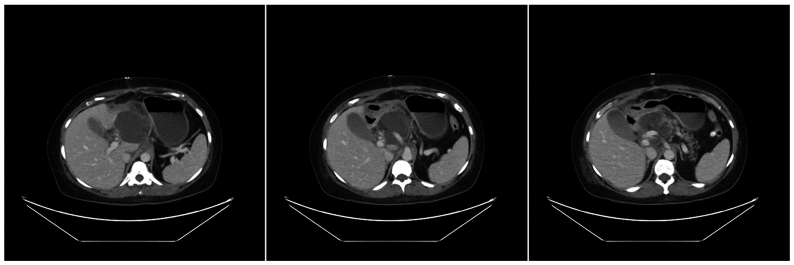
Axial cross sections of postoperative abdominal computed tomography (CT) scan with contrast shows peripancreatic fluid collection, involving portion of aortohepatic bypass graft.
